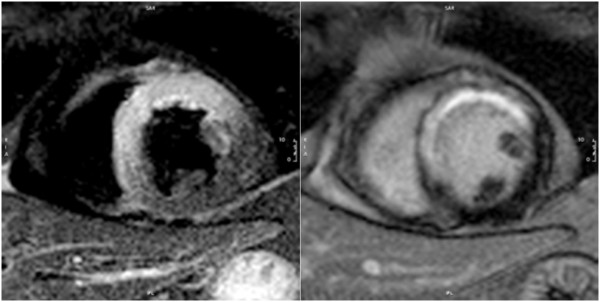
CMR in acute myocardial infarction. Acute reperfused infarction of the left anterior descending artery territory. Left: T2-weighted image (short-tau inversion recovery, STIR) in a midventricular short axis view with increased SI in the affected segments. Right: LGE image in the same orientation.
